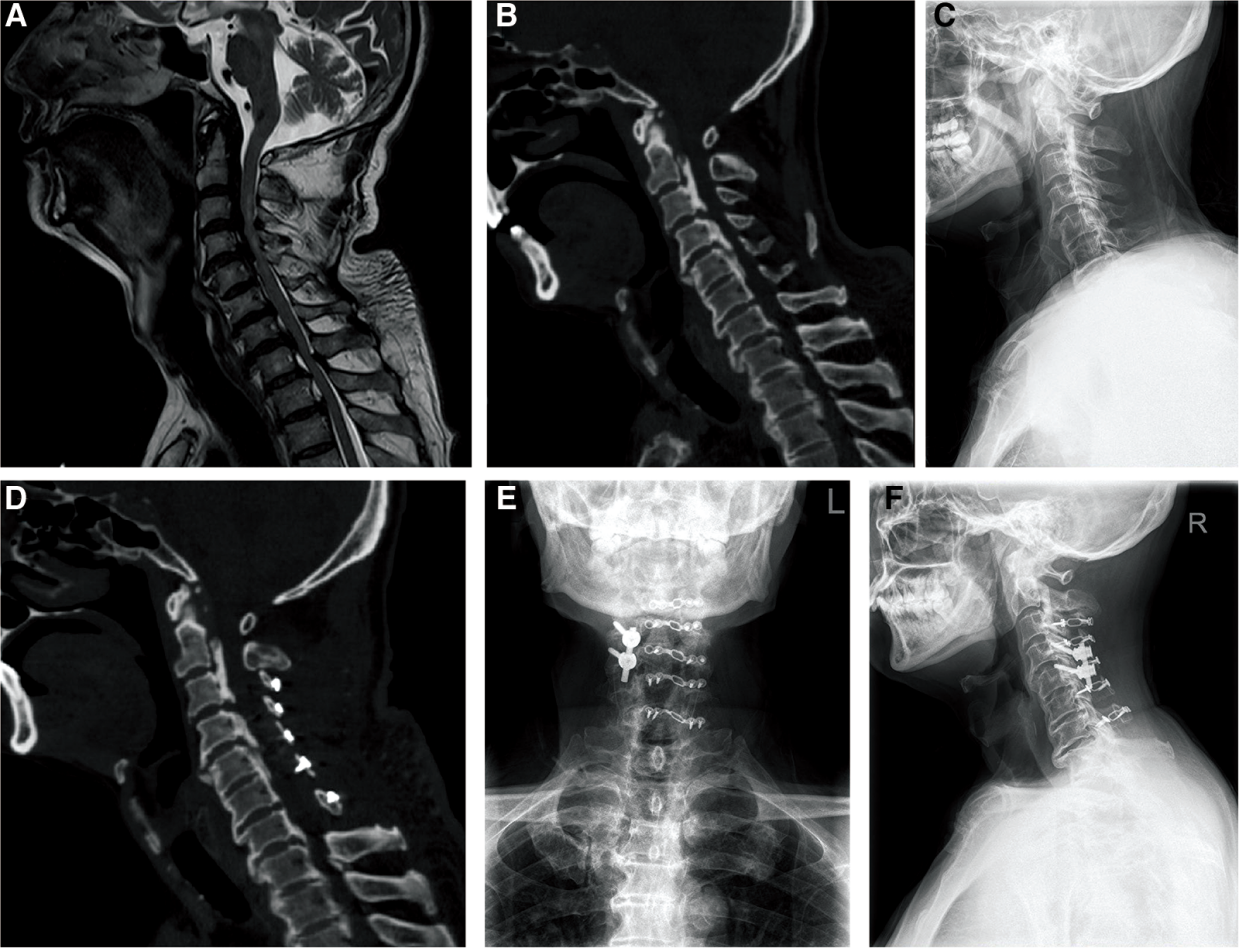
Imaging data of a typical case. A 66-year-old male patient with limb numbness caused by falling from height received single open-door laminoplasty and lateral mass screw fixation and fusion. (A) Preoperative MRI showed the rupture of C4/5 intervertebral disc and anterior longitudinal ligament, and the cervical spinal cord signal of C3–C6 increased on T2-weighted image. (B) Preoperative CT scan confirmed ossification of posterior longitudinal ligament and cervical spinal canal stenosis. (C) Preoperative radiograph. (D) Postoperative CT scan showed that the cervical spinal canal was enlarged and the effect of the operation was clear. (E,F). The anteroposterior and lateral radiographs 1 year after surgery suggested that the fixations were in good condition.
